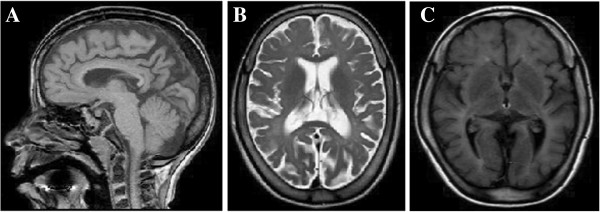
(A, B, C): Magnetic resonance imaging of Patient 1, diffuse hypomyelienation, thinning of the carpus callosum and moderate cerebral atrophy.
